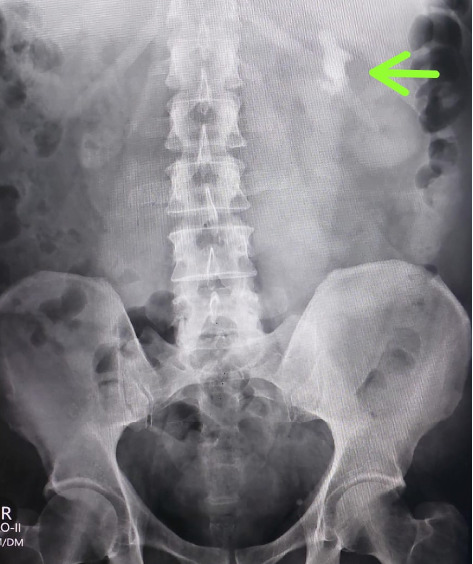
Pre-op x-ray showing left renal stone.
